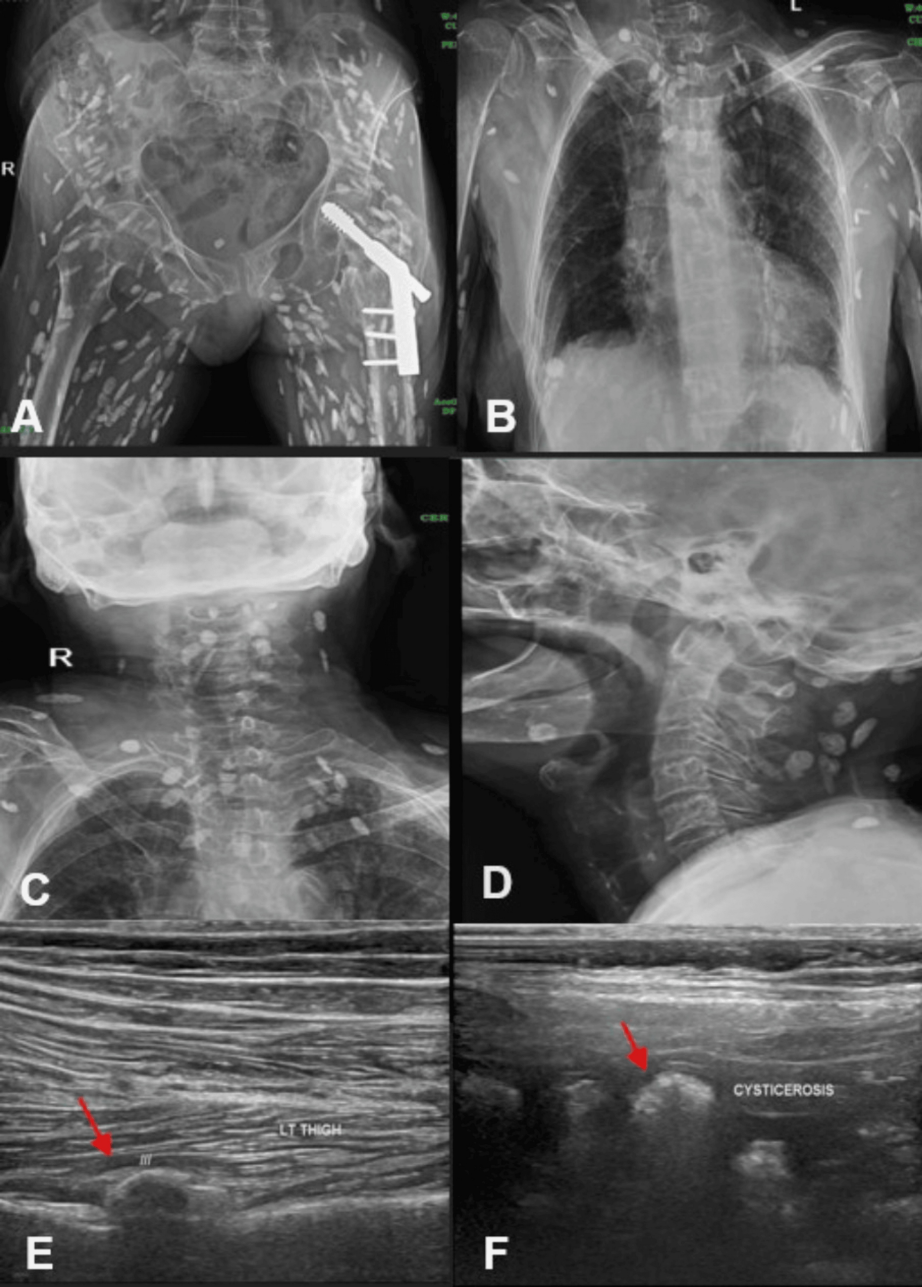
Radiographic and ultrasound images showing classic "rice grain pattern" of calcified cysticercosis Radiographic images (A-D) showing multiple typical rice grain appearances in the subcutaneous and muscular plane - suggestive of calcified cysticercosis. Ultrasound screening images of the thigh (E-F) showing multiple hyperechoic lesions with posterior acoustic shadowing (indicated by red arrows) - suggestive of calcified cysticercosis.
